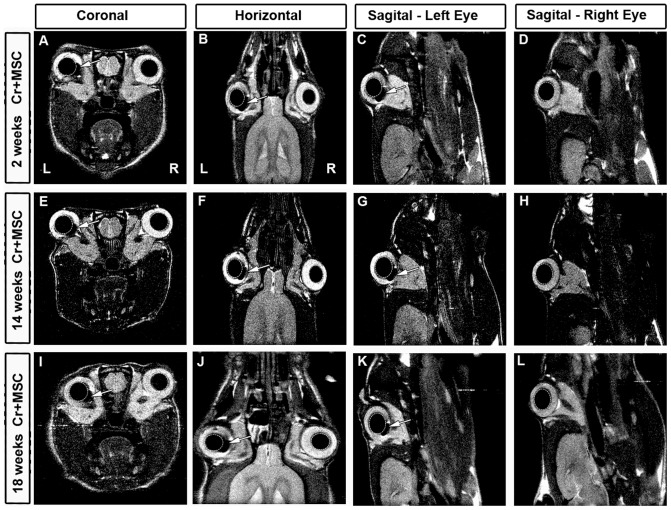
Detection of FeraTrack-labeled MSCs by *in vivo* MRI. MSC were labeled with Fera Track for 4 h and injected in the vitreous body of the left eye after optic nerve crush. (A-L) Representative images of *in vivo* MRI at coronal (A,E,I), horizontal (B,F,J), and sagittal planes (C,G,K; at left eye level and D,H,L; at right eye level) at different survival times. Arrows indicate hypointense (black) spots corresponding to FeraTrack-labeled cells in the vitreous body of the left eye. Labeled cells were found at 2 (A-C), 14 (E-G) and 18 weeks (I-K) after optic nerve crush and cell transplantation. The right eye was injected with the washing solution of the cells; no signal was detected (D,H,L and right hemisphere of coronal and horizontal images). The lenses are circled with dashed lines; right (R) and left (L) hemispheres.
